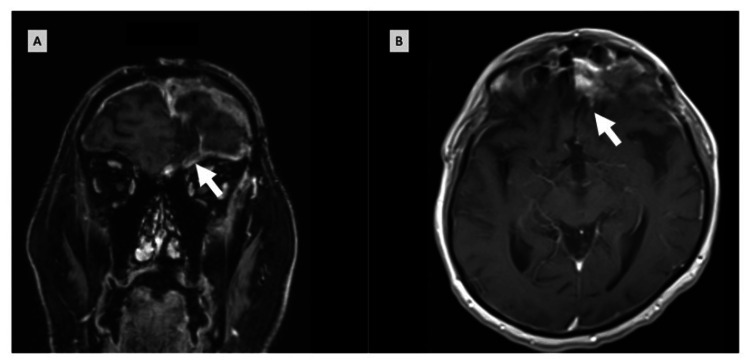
MRI five months after surgery, showing progressive enhancement in coronal (A) and axial (B) views.
